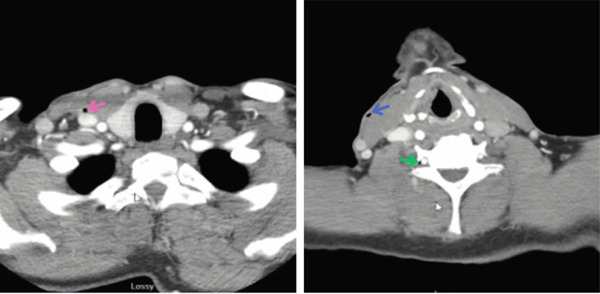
A CTA scan of the head and neck revealed air within the jugular venous system, as indicated by the pink arrow. A CTA scan of the head and neck revealed air in the track created by the central venous catheter under the skin (indicated by the blue arrow) and air in the vertebral venous system (indicated by the green arrow).
